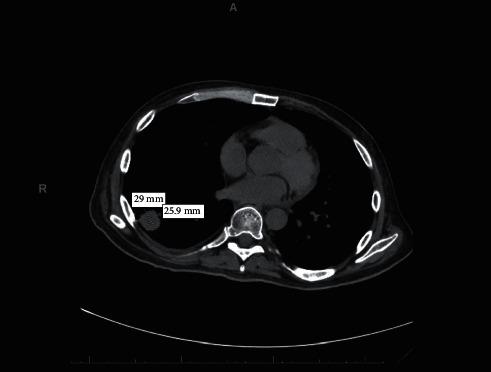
CT chest imaging showing increase in size of right lower lobe lung nodule.
